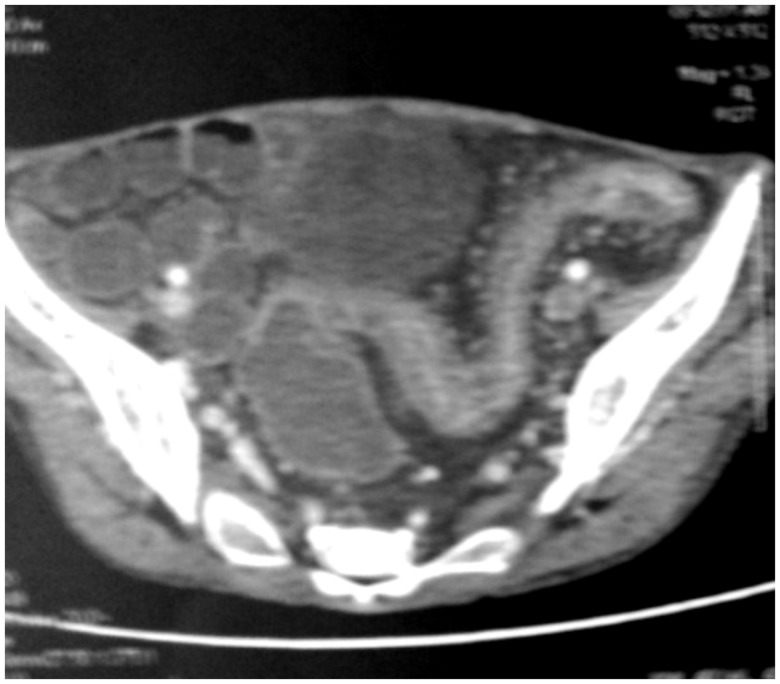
CT enteroclysis image, showing inflammatory thickening of the small intestine
